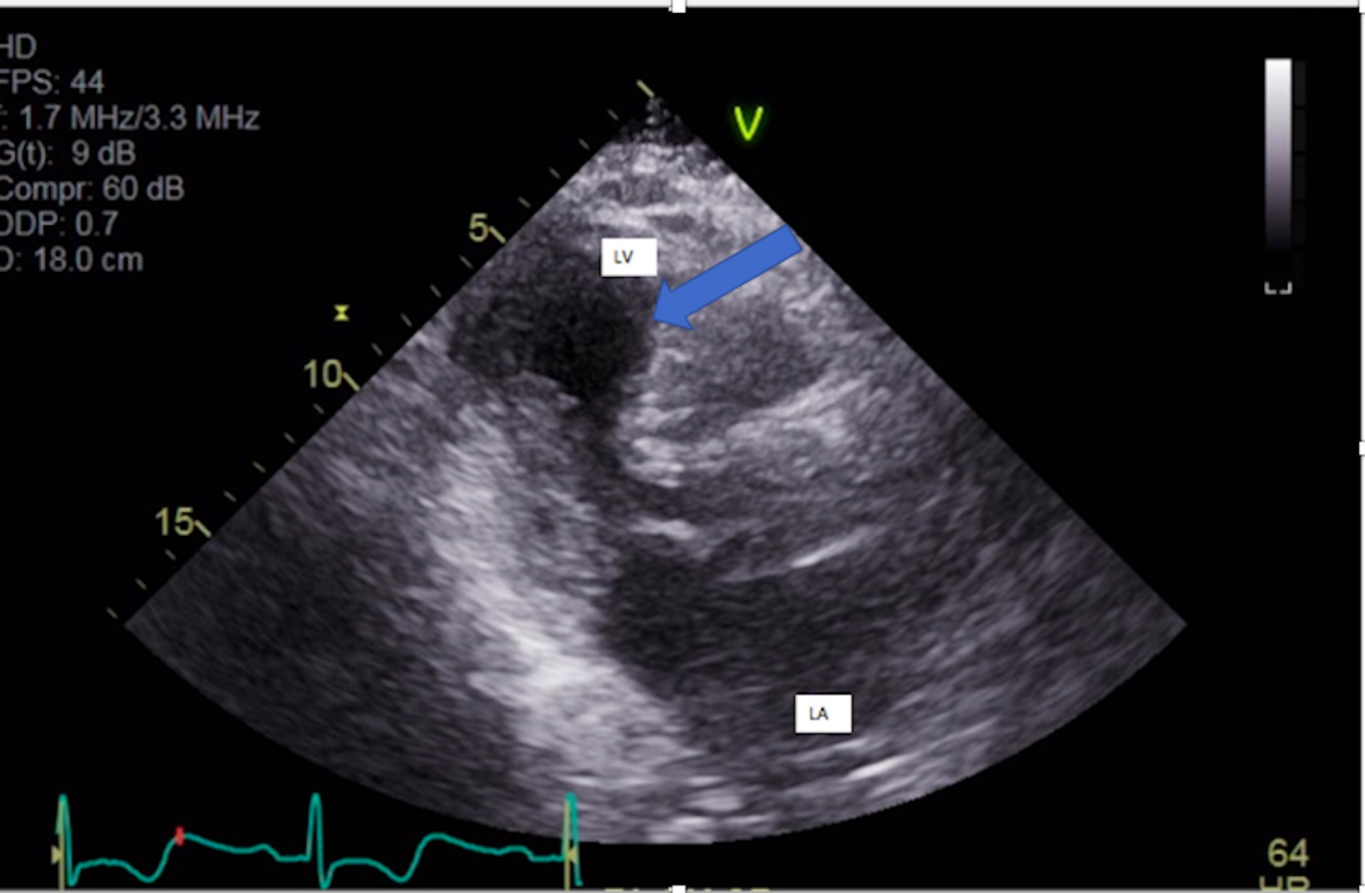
Parasternal long axis view showing apical ballooning due to hypokinesis of the apex, apical interventricular septum, and apical lateral wall LA: left atrium; LV: left ventricle
